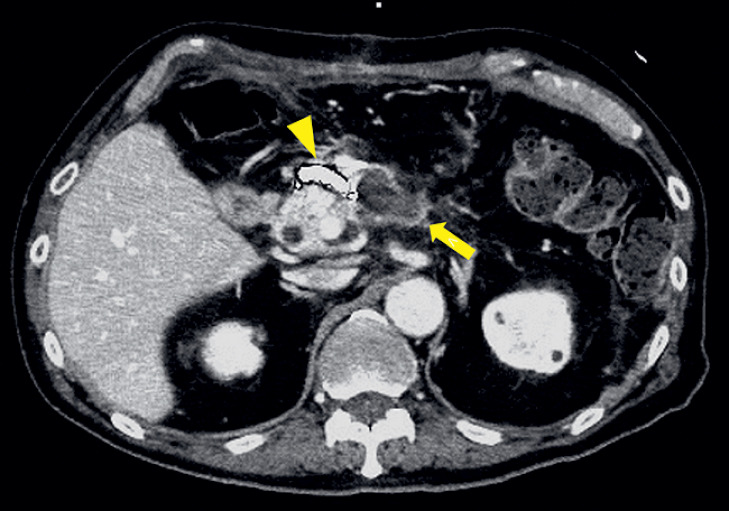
Contrast-enhanced computed tomography scan performed on postoperative day 27 showed a reduction in the size of the peripancreatic fluid collection (yellow arrow) and a coil for embolization in the right gastroepiploic artery (yellow arrowhead).
